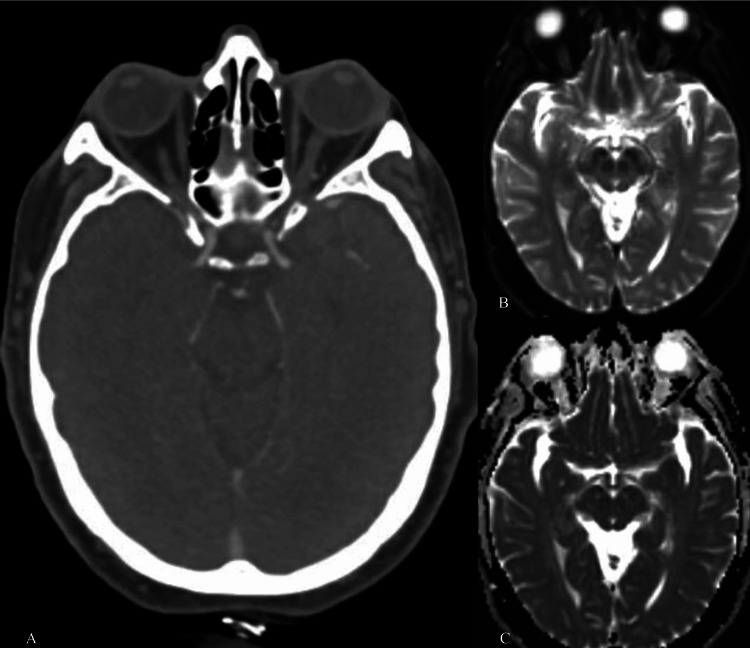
CT Brain, MRI Brain Diffusion-Weighted Imaging, MRI Brain Normal CT brain (A) obtained at the time of the suspected stroke. There is no evidence of bleeding. Additionally, an MRI brain with diffusion weighted (B) and apparent diffusion coefficient (C) imaging was obtained two days later. No evidence of diffusion restriction was identified indicating no ischemic damage. CT: Computed Tomography, MRI: Magnetic Resonance Imaging
